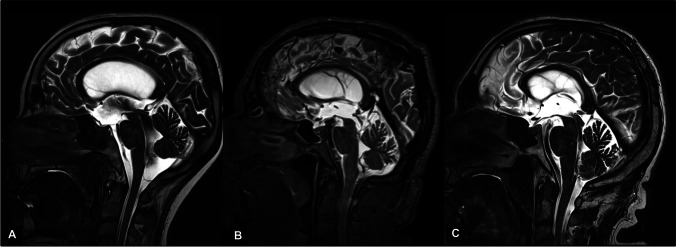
T2-weighted MRI in midsagittal view of the three different types of adult hydrocephalus analysed in our study is compared. A LOVA, distorted and empty sella, bulging of third ventricle floor, turbulent flow through the aqueduct and an enlarged cisterna magna with upwards shifted cerebellar vermis are showed. B LIAS, CSF flow throughout the aqueduct, which is totally obstructed by a thin septum, is absent. C iNPH, with a patent aqueduct, and no abnormalities in the anatomy of sella turcica, third ventricle floor and cisterna magna
